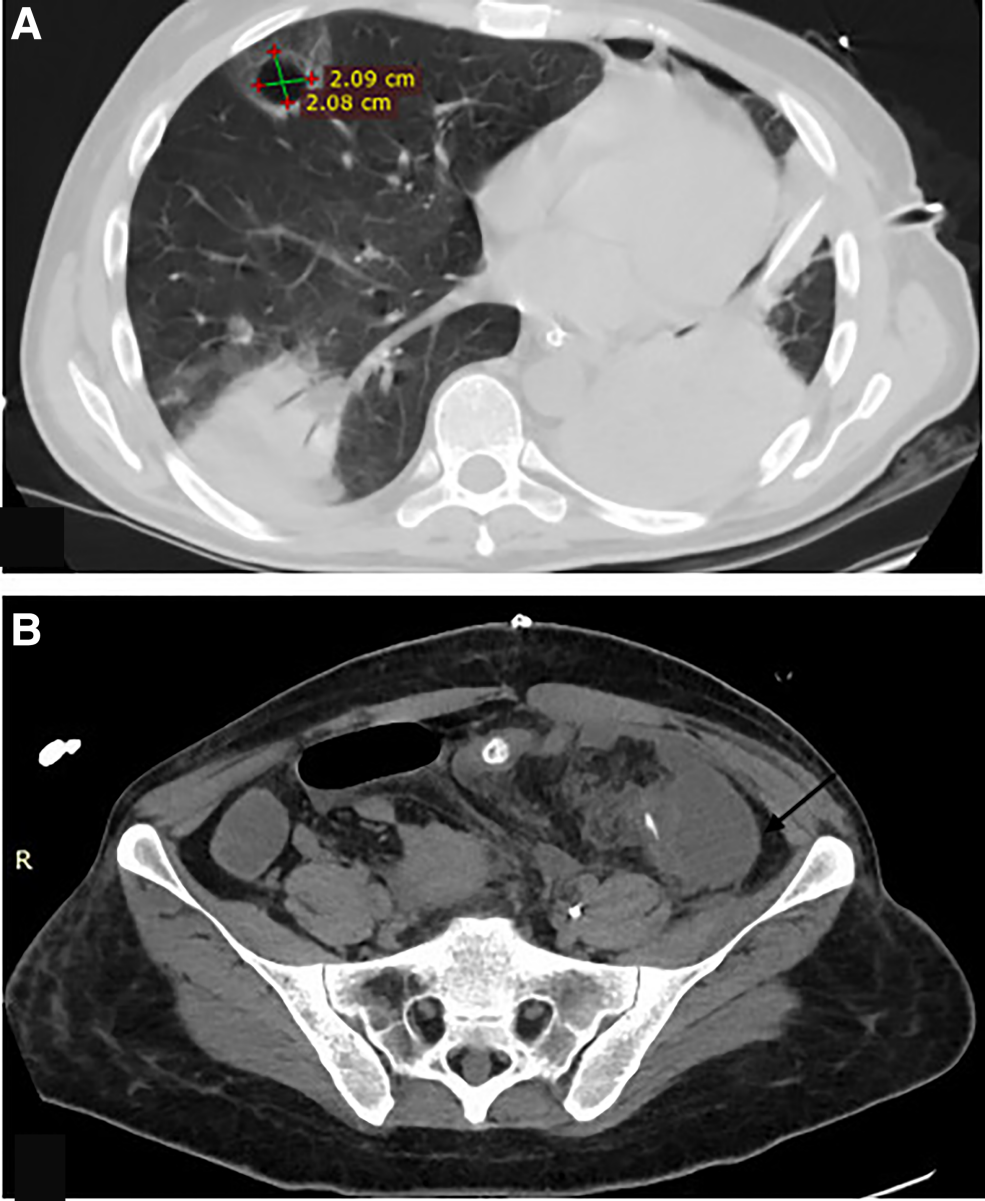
(A) Chest -Ct scan revealing a right upper lobe cavitary lesion surrounded by ground glass opacities, measuring about 2 × 2 cm.(B) Abdomen CT scan intraabdominal fluid collections at the left paracolic getter measuring about 4.5 × 4.5 × 10 cm (arrow) with adjacent smaller one measuring about 3 × 4.5 × 5 cm.
